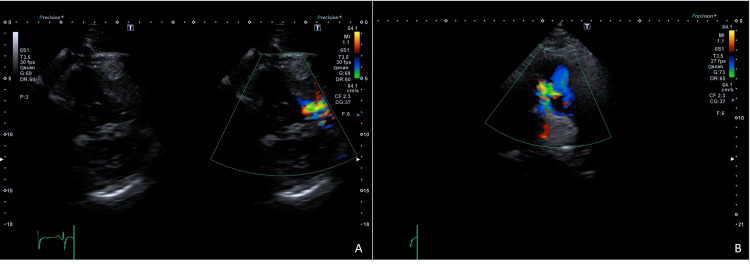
Interventricular communication by color Doppler echocardiography. (A) Parasternal short-axis view at the basal level and large vessels. (B) Systolic left-to-right flow jet.
